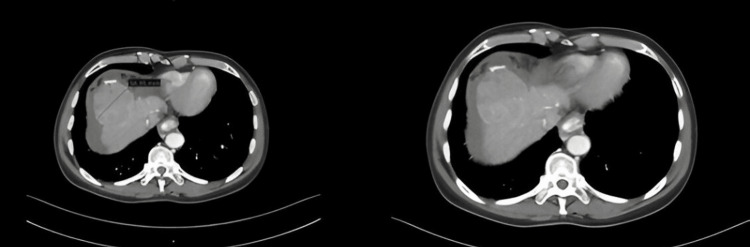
CT abdomen with contrast Showing a large liver lesion in a background of liver cirrhosis, concerning for malignant liver tumors
